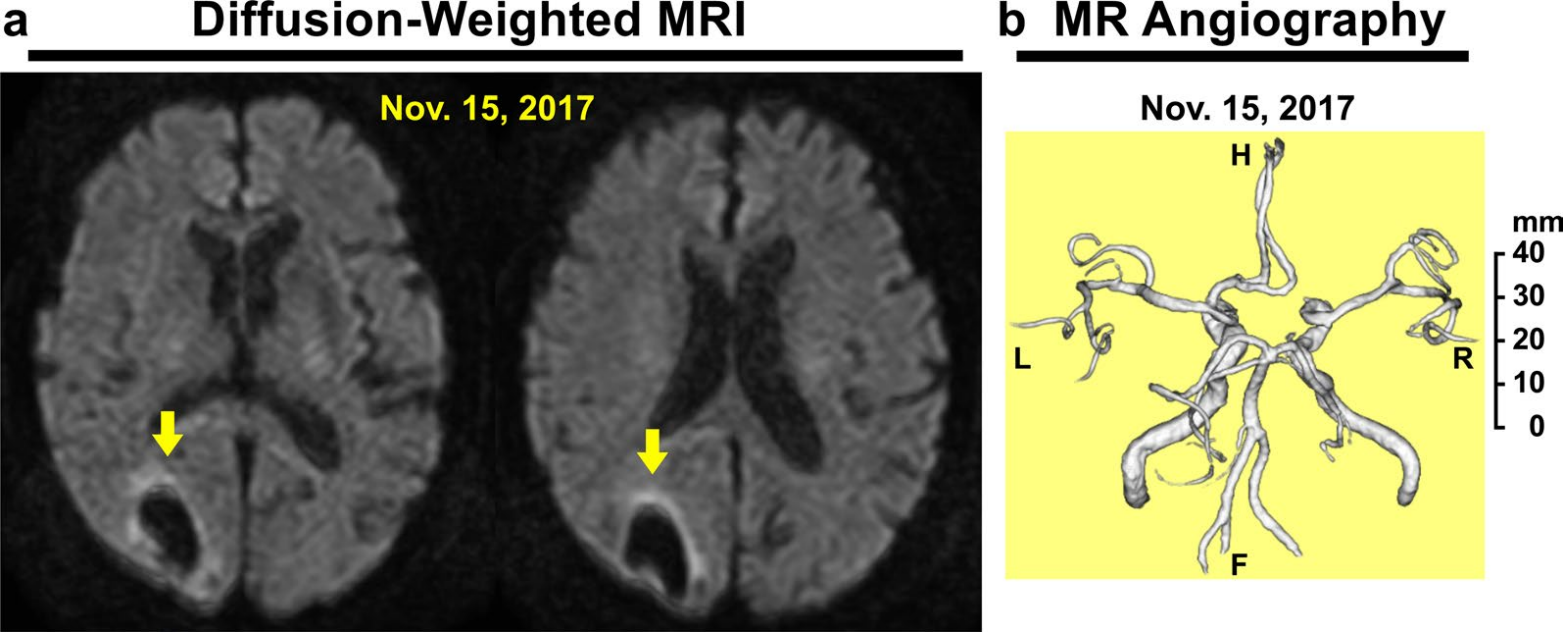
Diffusion-weighted magnetic resonance imaging (MRI) (a) and magnetic resonance (MR) angiography (b) of the brain on 15 November 2017. Two different slices were included (a). Diffusion-weighted MRI revealed the low-intensity area in the same region of the right occipital lobe (a yellow arrowheads); MR angiography demonstrated no vascular anomalies or malformation. *H* head, *F* feet, *R* right, *L* left (b)
